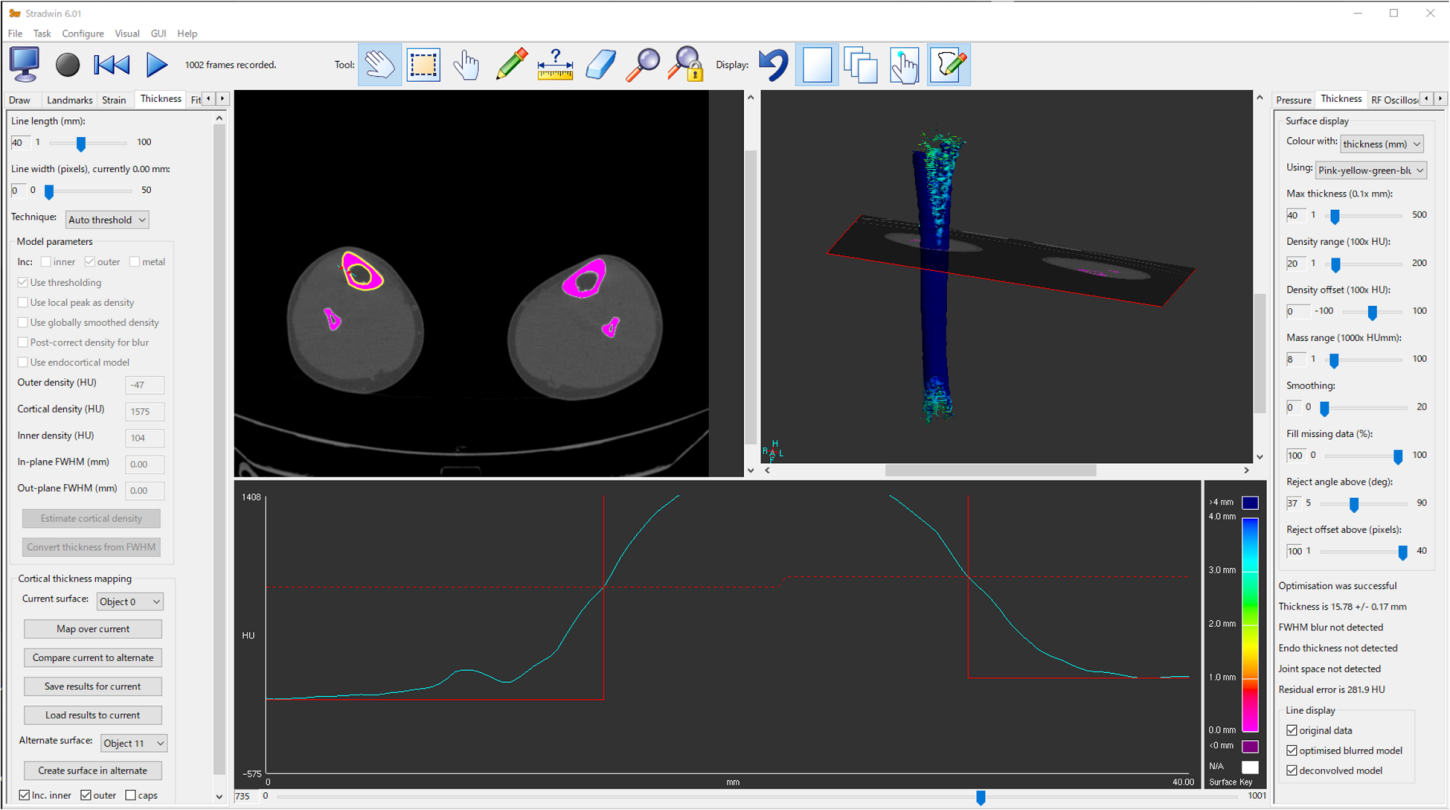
Automatic calculation using the high-resolution cortical thickness measurement from clinical CT data. The technique relies on a mathematical model of the anatomy and imaging system, which is fitted to the data at a large number of sites around the femur. Given prior segmentation of the tibial diaphysis, CT values are examined along short lines that straddle and are perpendicular to the cortex
